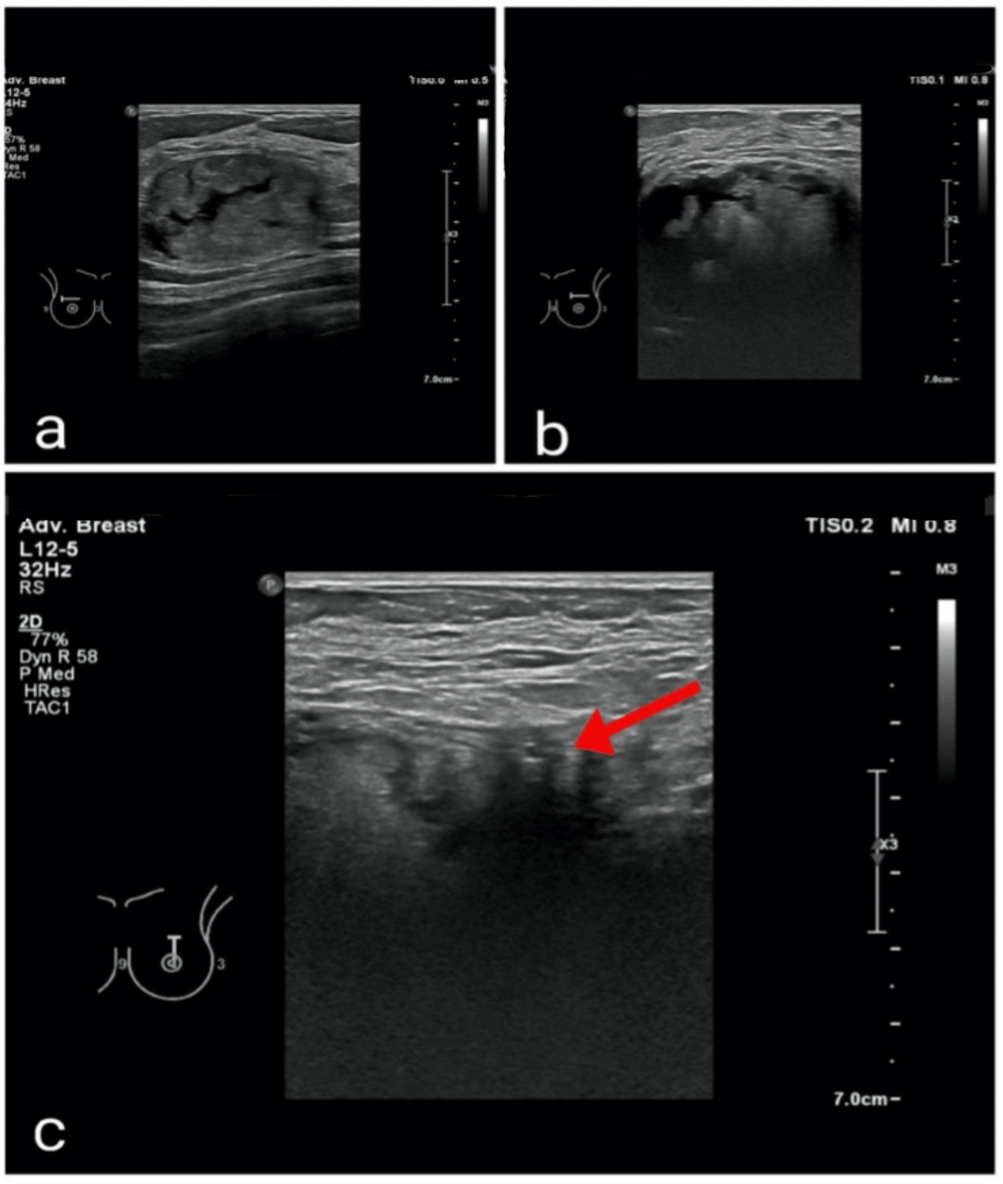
Pre-lumpectomy ultrasound Ultrasound images showing bilateral complex cystic and solid masses at the 12:00 position (a, b), with features suggestive of oil cysts or fat necrosis. In the left breast (c), an irregular mass with architectural distortion is observed adjacent to the area of fat necrosis (see arrow).
